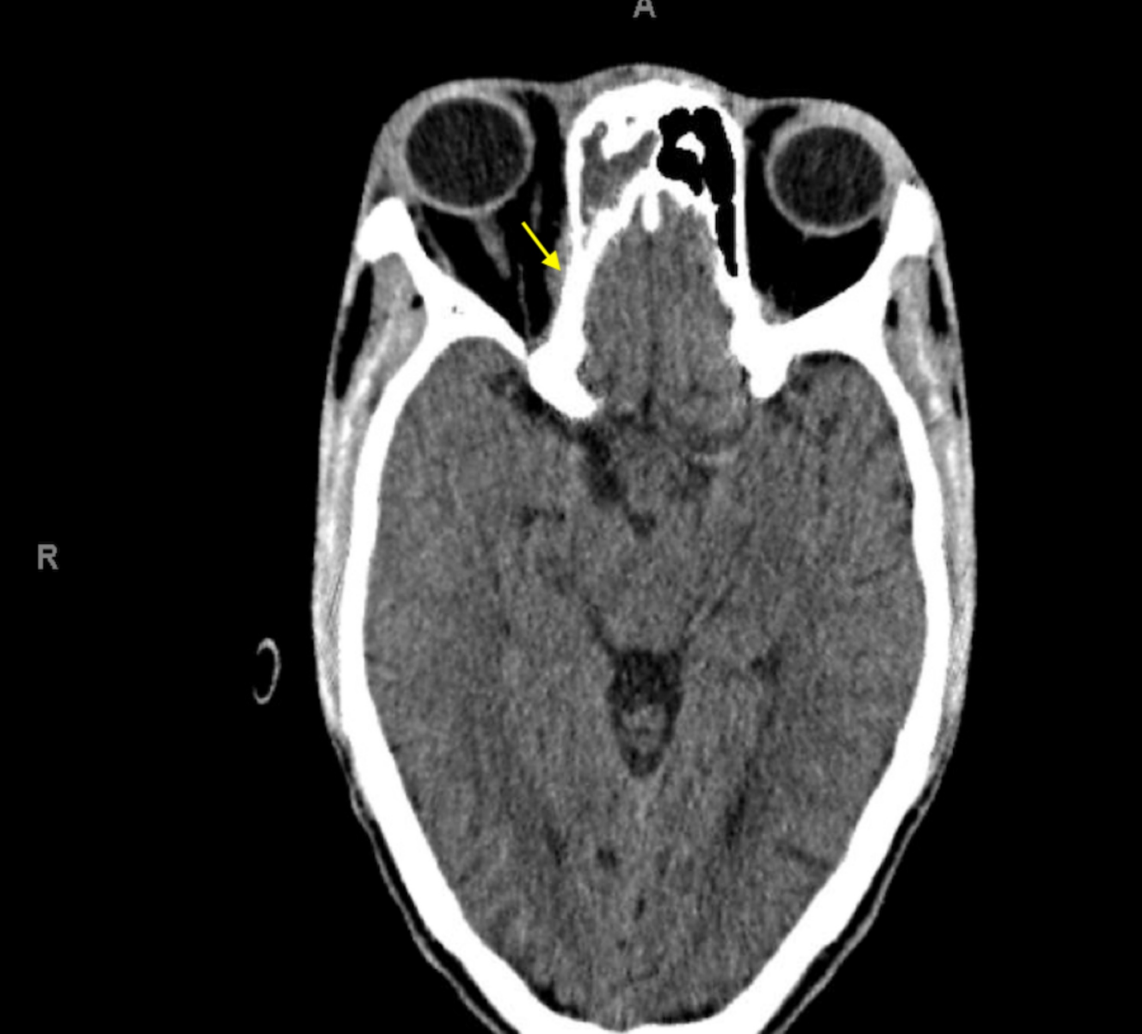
Opacification of the right ethmoid sinus on computed tomography of the head. Yellow arrow points to mild subperiosteal inflammation, which could be the beginning stages of a subperiosteal abscess.
